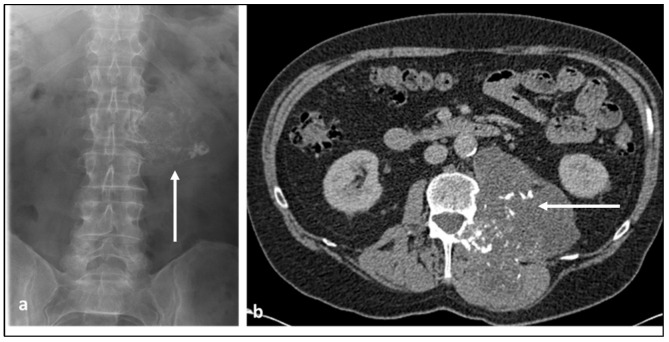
AP radiograph of the lumbar spine (a) and axial CT of the abdomen (b) demonstrating a chondrosarcoma arising from the left lateral aspect of the L2 vertebra, involving the body, left transverse process and pedicle (white arrows). Large soft tissue component with internal “rings and arcs” type calcification, in keeping with a chondroid matrix, is a characteristic feature.
